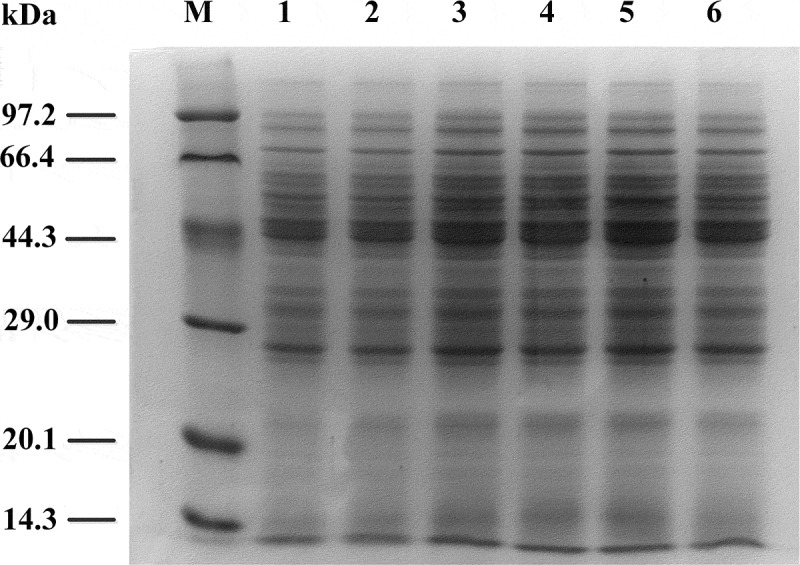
SDS-PAGE analysis of *E. coli* TRTH after ultrasonication. M: Low molecular weight protamine (LMWP) marker. Lanes 1 and 2: 5-μL samples. Lanes 3 and 4: 10-μL samples. Lanes 5 and 6: 15-μL samples.
